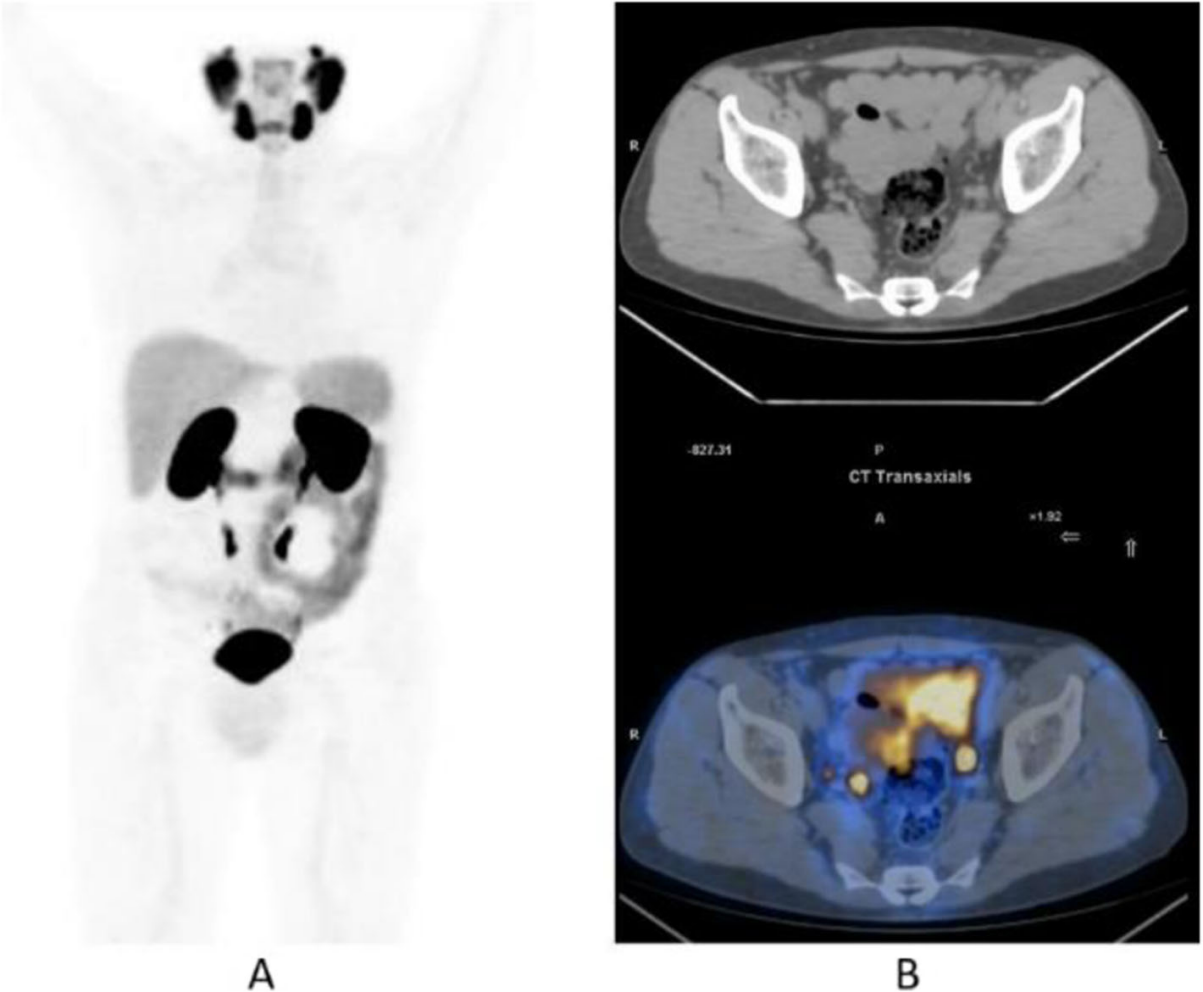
^68^Ga-PSMA, prostate cancer, biochemical recurrence (BCR): sensitivity. *Clinical history*: 64 y.o. man with prostate cancer, radical prostatectomy as primary treatment Gleason score 4 + 4; PSA = 0.7 ng/ml, PSA dt 6 months, TTR 12 months, candidate for salvage radiation therapy in the prostatic fossa. *PET/CT findings*: single focus of increased uptake seen in a right external iliac lymph node (obturatory) measuring 8 mm in maximum diameter. a MIP; b CT and fused images
